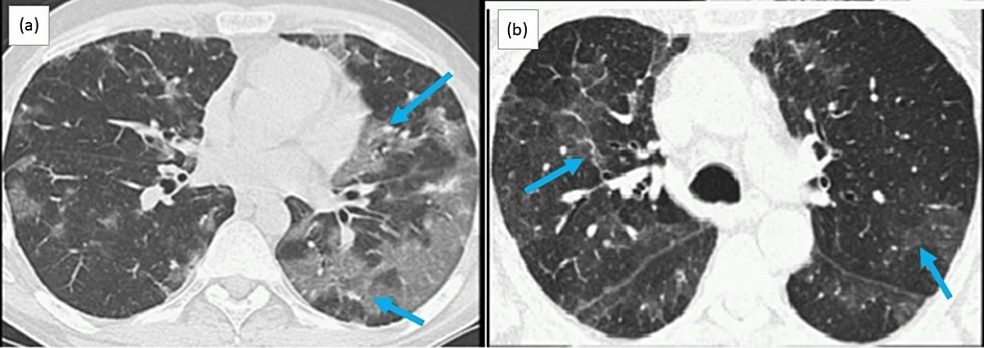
High-resolution computed tomography of the chest demonstrating diffuse crazy-paving and consolidation bilaterally (a) and focal parenchymal band in both lungs (b).
